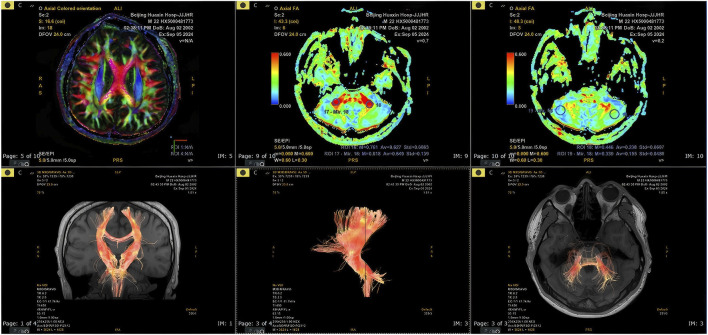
MRI-Brain DTI showed that the bilateral corticospinal tracts were normally aligned, without obvious interruption or displacement. Cerebellar fiber tracts were slightly reduced. Fractional anisotropy (FA) values indicated that the FA of the right cerebellar hemisphere was slightly lower than normal (normal range: ≥0.18). SPECT cerebral perfusion imaging revealed mildly heterogeneous blood flow in the frontal and parietal lobes.
